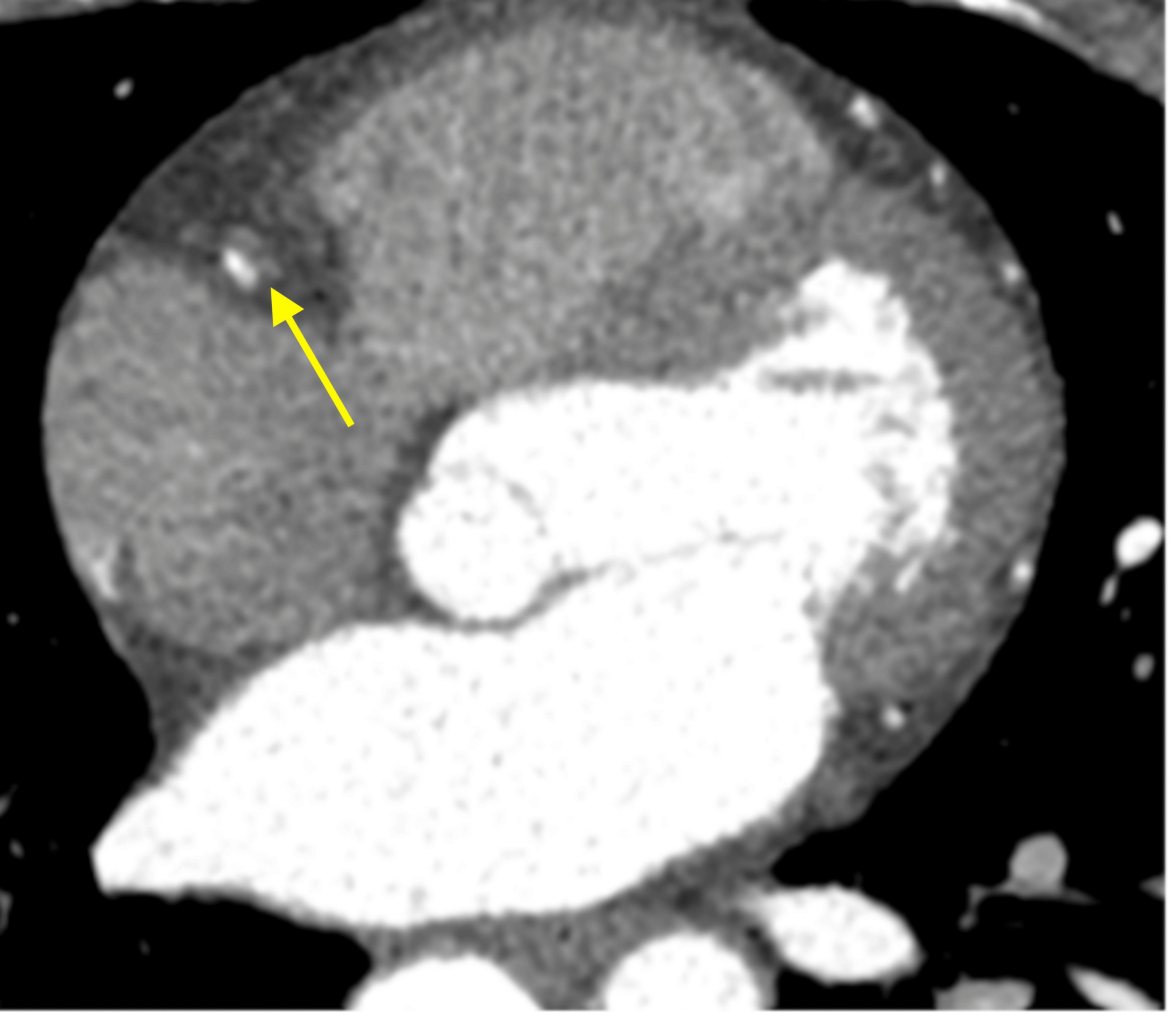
Axial view of SCAD (yellow arrow) in the RCA on CCTA SCAD: spontaneous coronary artery dissection; RCA: right coronary artery; CCTA: coronary computed tomography angiography
